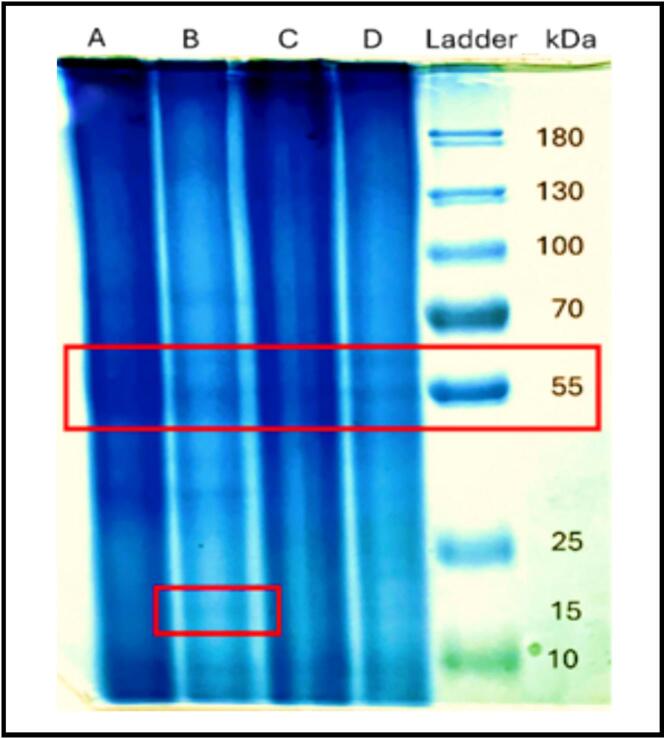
SDS-Page of BLP obtained by conventional alkaline extraction and ultrasound-assisted alkaline extraction at different concentrations: A: 10 mg BLP after CAE, B: 5 mg BLP after CAE, C: 10 mg BLP after UAAE, D: 5 mg BLP after UAAE.
